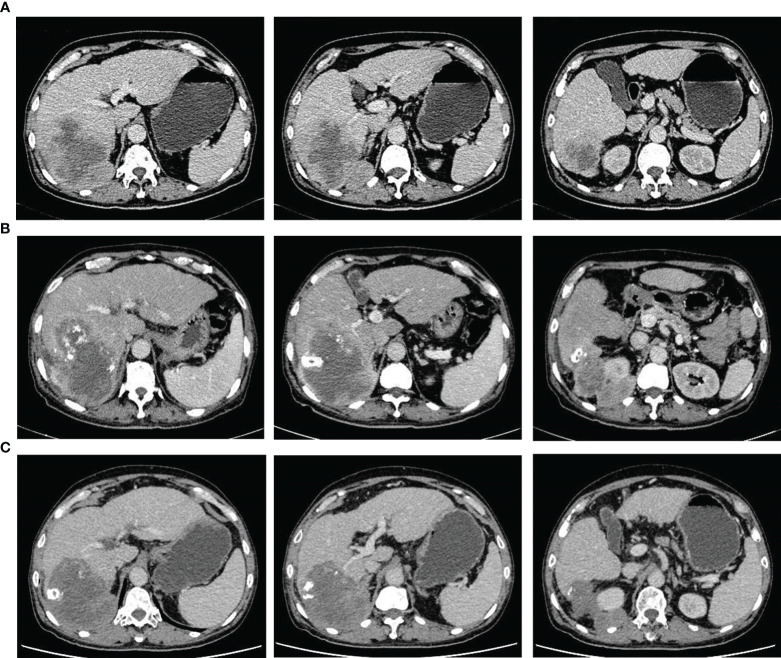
Representative computed tomography images showing changes in the tumor throughout the treatment period prior to surgery. (A) perihepatic tumor with blurred edges in the right liver at initial diagnosis. (B) extrahepatic tumor growth (with a blurred boundary) covering the upper pole of the right kidney, 2 months after transcatheter arterial chemoembolization. (C) stable tumor size and clear boundary after treatment with tyrosine kinase inhibitors and anti-PD-1 antibodies for 6 months.
